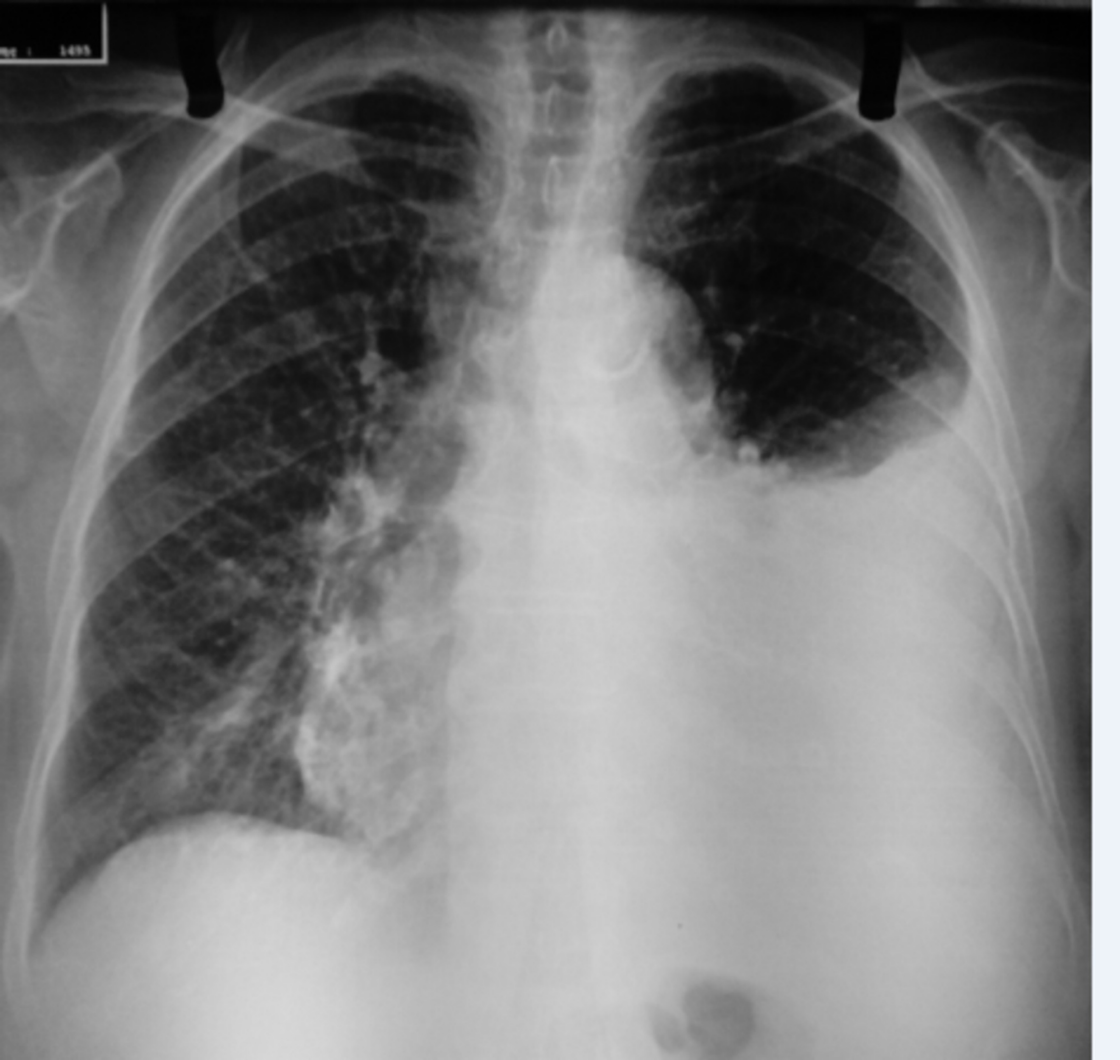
Chest X-ray on admission showing a large left pleural effusion
